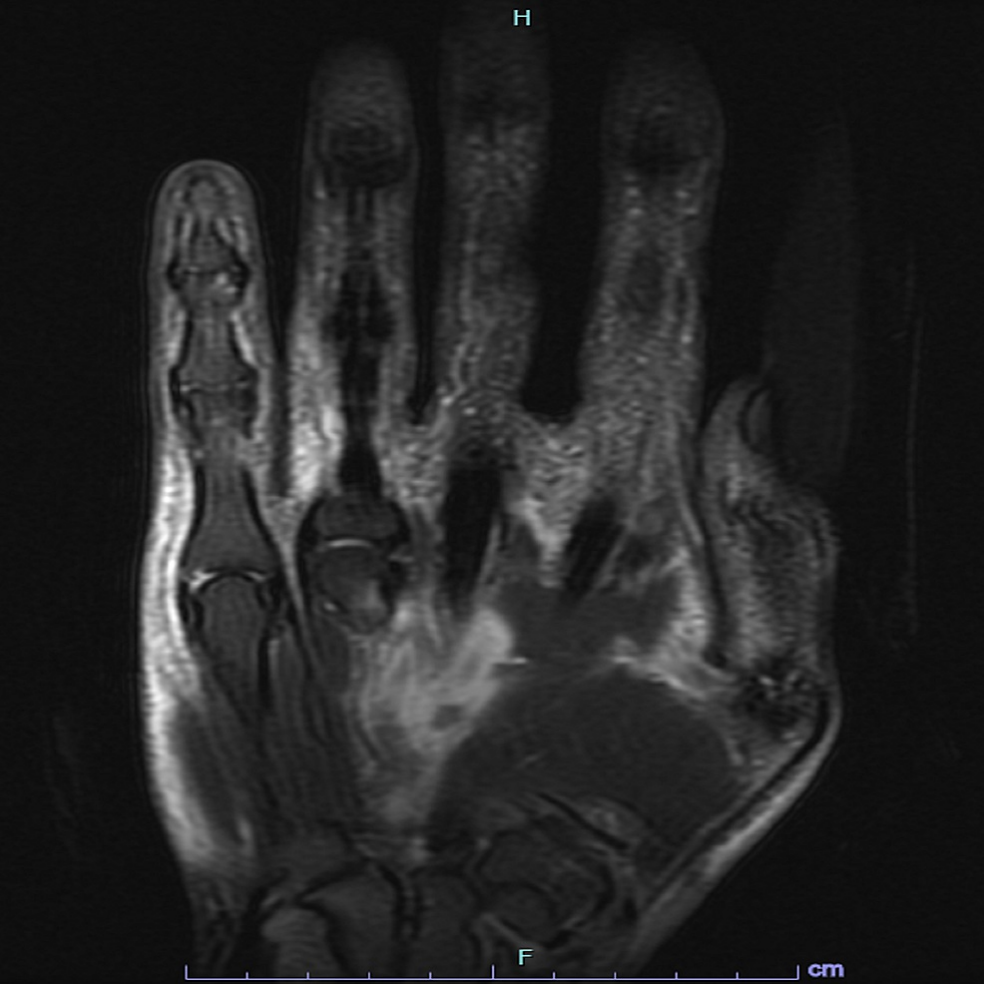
MRI of the right wrist without contrast The image is showing dorsal subcutaneous edema throughout the hand and engulfing the dorsal tendon sheaths.
